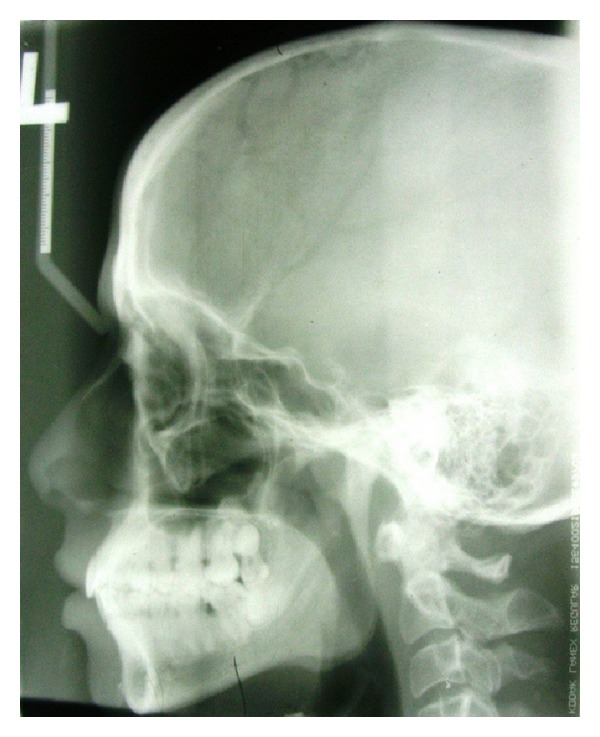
Plain radiograph revealing no bony involvement, but phleboliths can be noticed.
